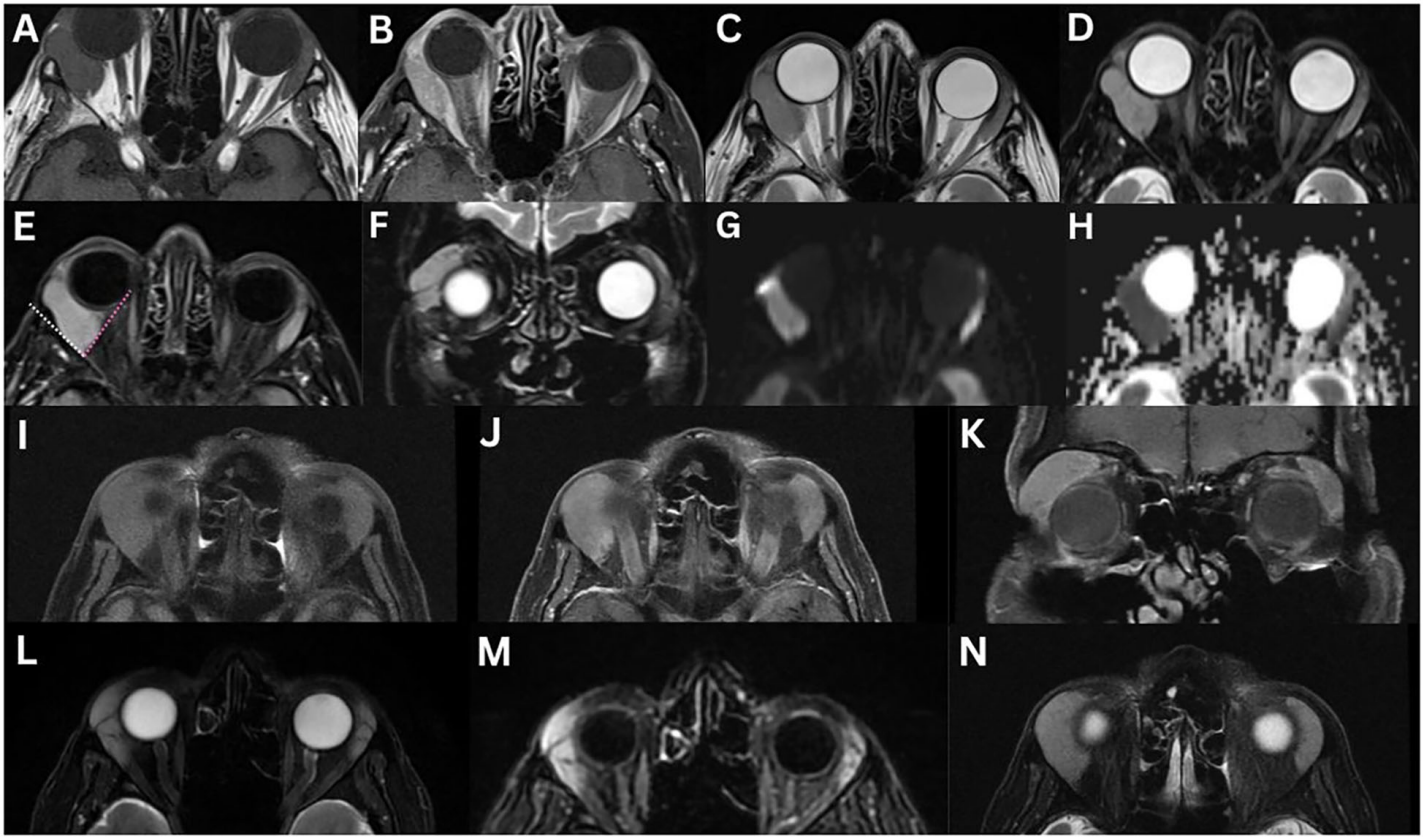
Marginal lymphoma of the right lacrimal gland (A–H). MRI T1-weighted axial (A), axial fat-suppressed contrast-enhanced (B), T2-weighted axial (C), axial fat-suppressed (D), axial FLAIR (E), and T2-weighted coronal fat-suppressed (F) sequences demonstrate a well-defined homogeneous mass that is isointense on T1-weighted imaging, and hyperintense on T2-weighted imaging, relative to extraocular muscles. The mass moderately enhances following contrast administration. There is evidence of a lateral wedge sign (B). The posterior angle between the lacrimal gland (pink dotted line) and lateral orbital wall (white dotted line) is acute (E). There is restricted diffusion on axial DWI (G) and ADC (H). Mantle cell lymphoma involving the lacrimal glands (I–N). MRI T1-weighted axial fat-suppressed FLAIR (I) and fat-suppressed contrast-enhanced axial (J) and coronal (K), T2-weighted axial (L), axial fat-suppressed FLAIR (M), and axial T2-weighted fat-suppressed (N) sequences demonstrate asymmetrical bilateral enlargement of the lacrimal glands, more pronounced on the right. The right lacrimal gland, confirmed by biopsy to be mantle cell lymphoma, demonstrates a homogeneous intermediate intensity signal on T1-weighted imaging and a high intensity signal on T2-weighted imaging. There is homogeneous enhancement with contrast administration.
